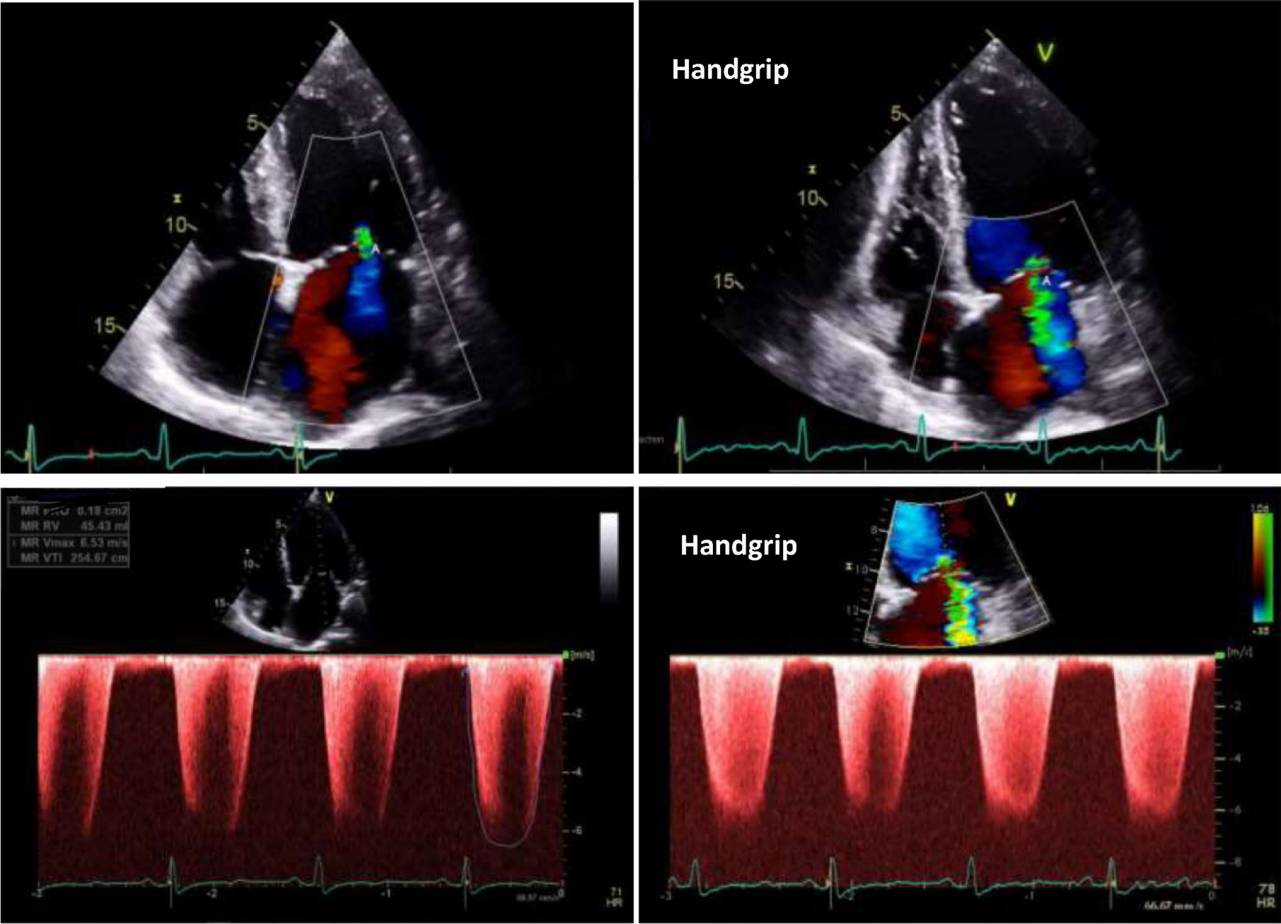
Baseline echocardiographic apical 4-chamber view with color Doppler (upper left) with continuous-wave Doppler assessment (lower left) in a patient with secondary mitral regurgitation (MR). There was an increase of MR (upper right) with denser continuous-wave Doppler signal during handgrip exercise (lower right)
